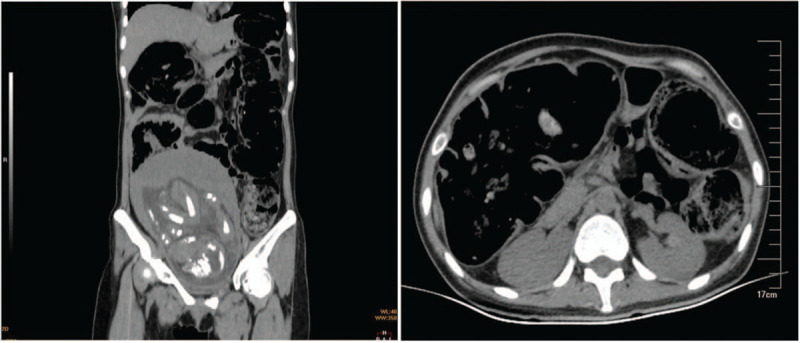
Pre-surgery CT scan. The colon is distended.
